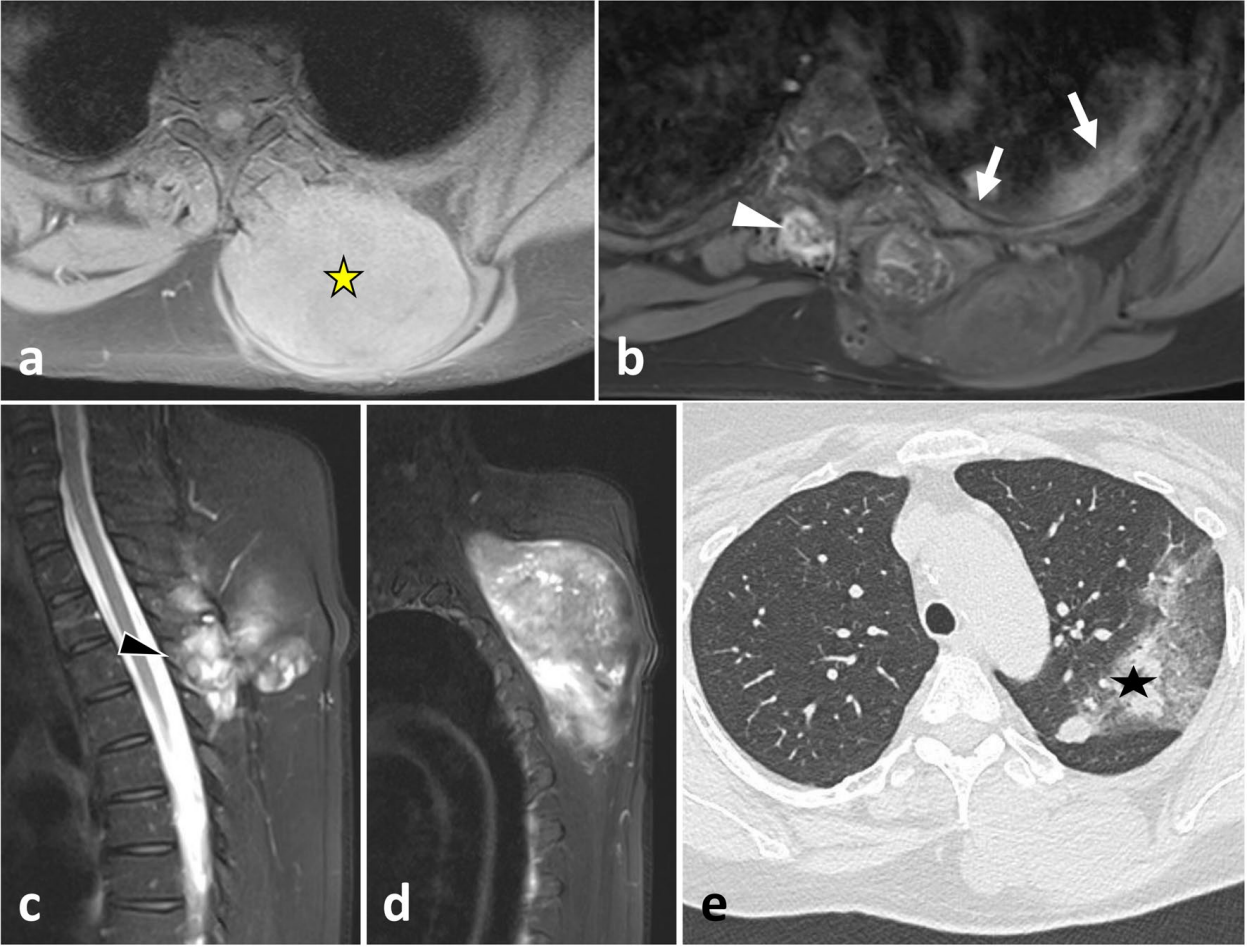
Undifferentiated sarcoma* of left thoracic paraspinal soft tissues in a 42-year-old female with severe non-mechanical thoracic pain and palpable hard mass. Axial fat-suppressed T1WI (a) and gadolinium-enhanced T1WI (b) show a necrotic mass in bilateral hypaxial muscles. Sagittal STIR WI (c, d) shows paraspinal extension along five segmental levels. Sagittal T2WI shows bone inflammation of the spinous process (black arrowhead). Axial fat-suppressed T1WI and axial CT scan (e) demonstrate tumour’s spread towards the opposite side (white arrowhead), pleural extension (white arrows) and pulmonary extension (star). The yellow star shows the epicentre of the mass
